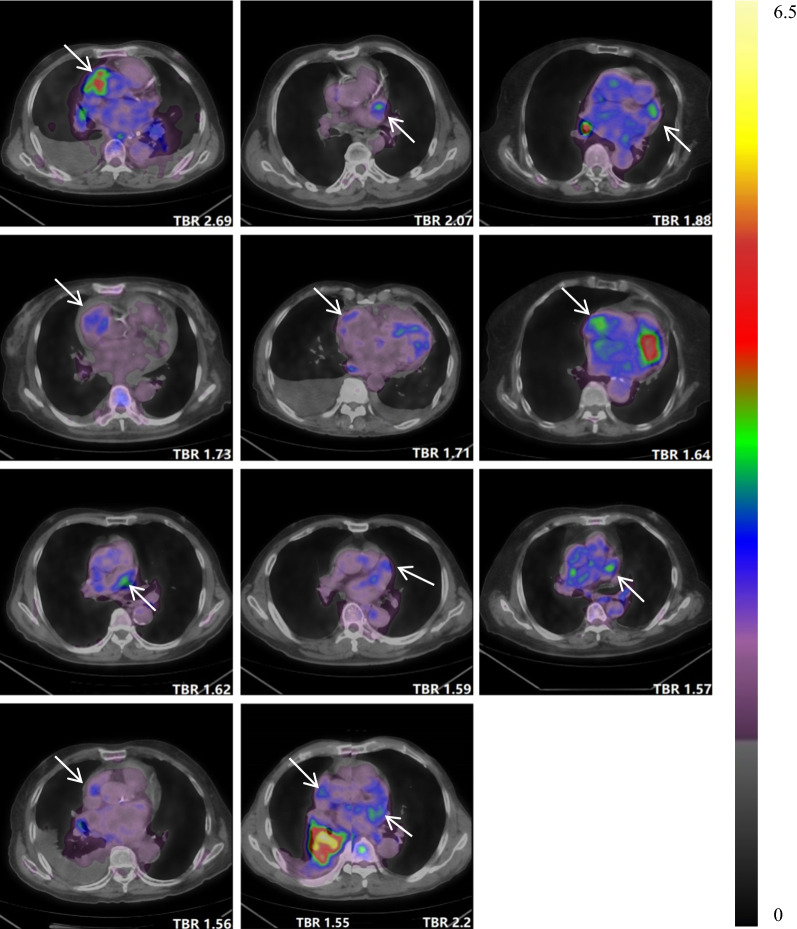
Axial positron emission tomography/computed tomography (PET/CT) images of 11 atrial fibrillation (AF) patients with increased uptake in the atrial appendage (arrows indicate abnormal uptake)
